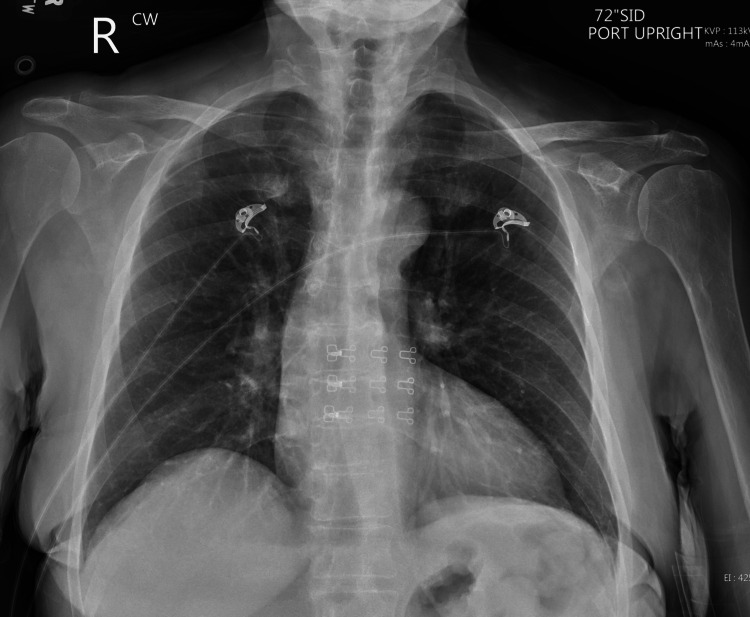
Chest radiograph following thoracentesis Resolved bilateral pleural effusions after diagnostic and therapeutic thoracentesis for the evaluation of pleural fluid
